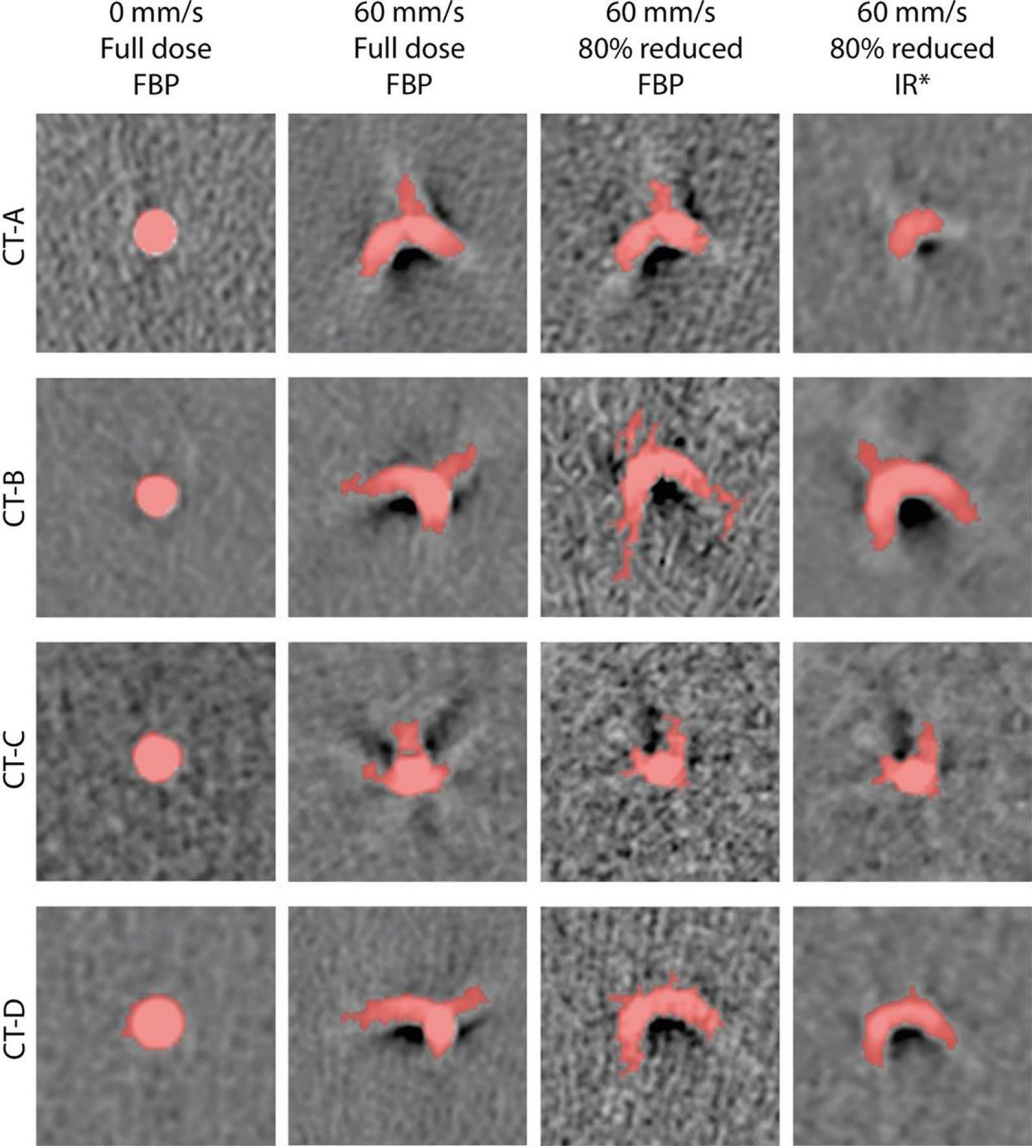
Representative images of the motion artifacts for all CT systems at all velocities, clinical full radiation dose and FBP. Window center was 90 HU and window width was 750 HU. *For each CT system. *FBP* filtered back projection;
